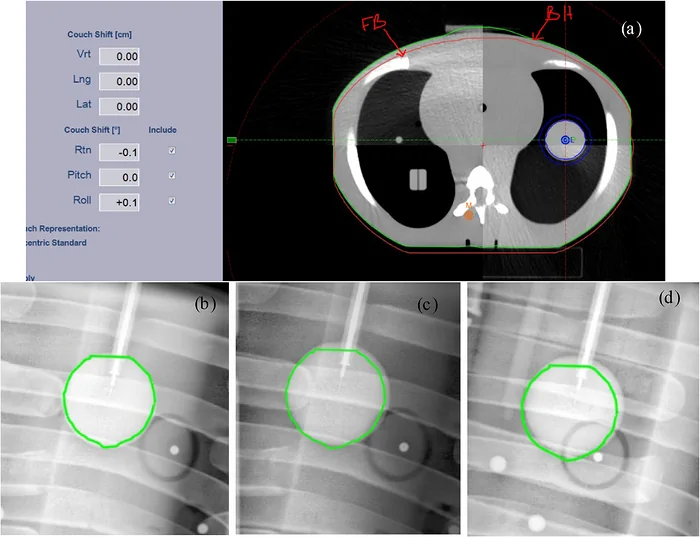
(a) Post‐SGRT CBCT verification of the BH setup prior to SITI delivery. Green and red contours = planning BH external and copied FB external; the offset reflects the A–P displacement coupled to the platform's S–I motion via the inclined wedge. Registration yielded 0.00 cm translations and rotations ≤0.1°, confirming negligible residual setup error. (b–d) Triggered kV images for (b) SITI delivery, (c) SITI + 1 mm induced shift, and (d) SITI + 2 mm induced shift, with the verification volume (green; tumor contour, x = 0 mm) overlaid on each live image. Panels (c) and (d) show the tumor deviating from the verification volume due to the induced shifts.
